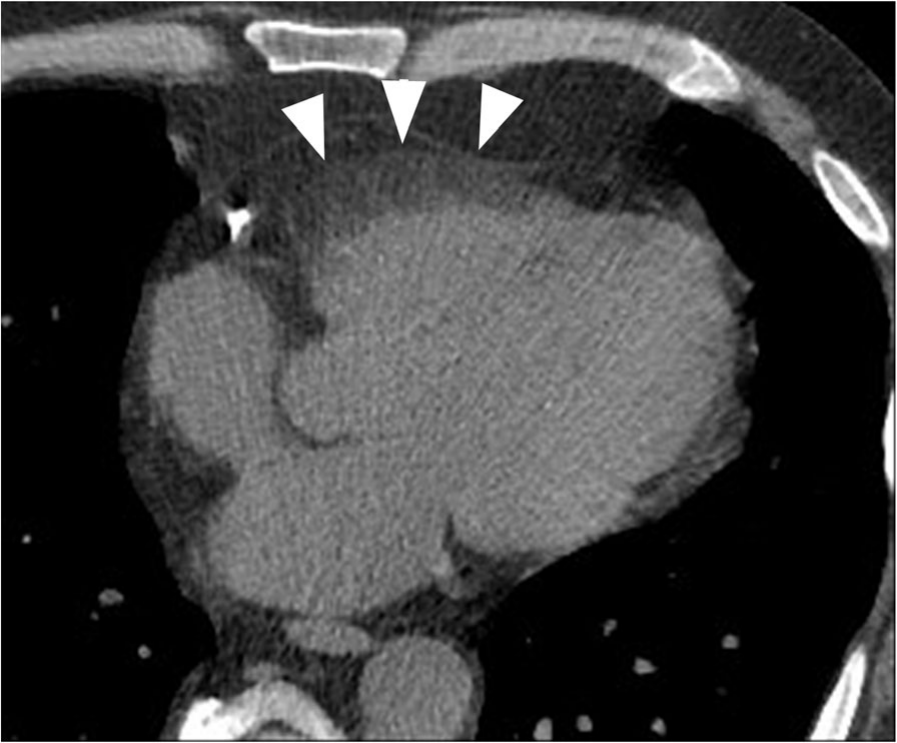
Axial non-contrast CT (which was performed to follow up a lung nodule) demonstrates fat replacement in the free wall of the right ventricle (white arrowheads) in a 50-year-old male
